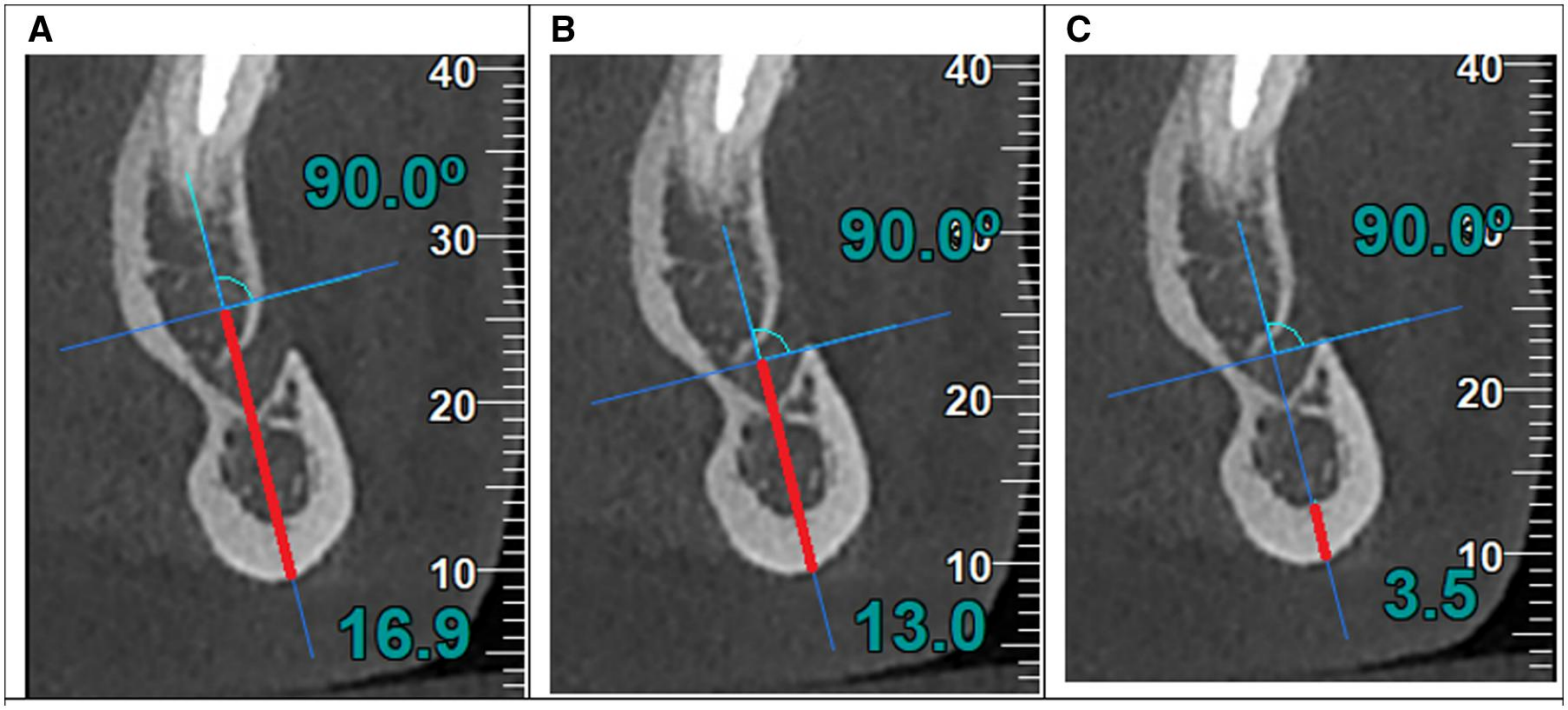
The measurements of S, I, and W indices on coronal CBCT images. (A) S, the distance from the superior margin of the MF to the inferior border of the mandible. (B) I, the distance from the inferior margin of the MF to the inferior border of the mandible. (C) W, the inferior cortical width of the mandible. The indices are the following: CTI(S) = W/S, CTI(I) = W/I, CTMI = W.
